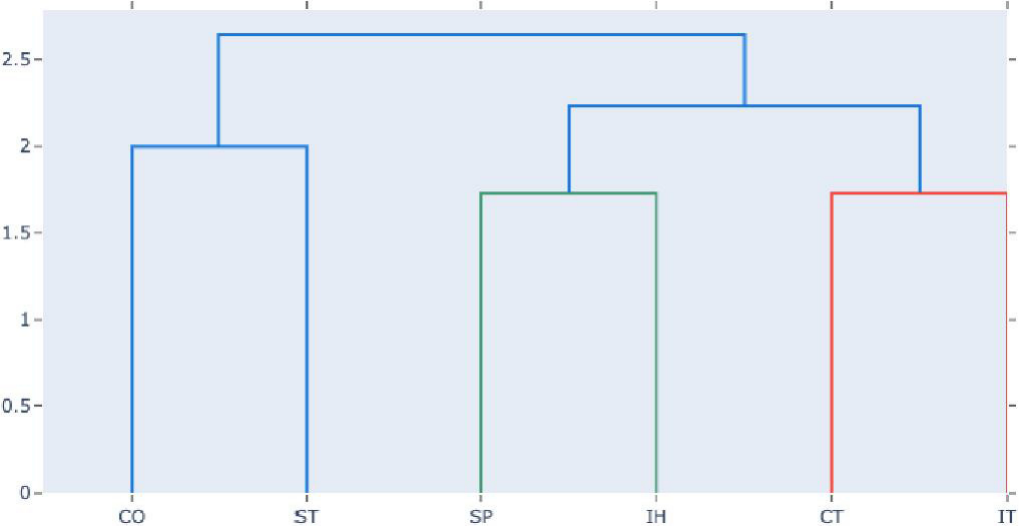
Relationship between role-stereotypes based on the common co-occuring design smells. The role-stereotypes are abbreviated as follows; Coordinator (CO), Structurer (ST), Service Provider (SP), Controller (CT) and Interfacer (IT).
